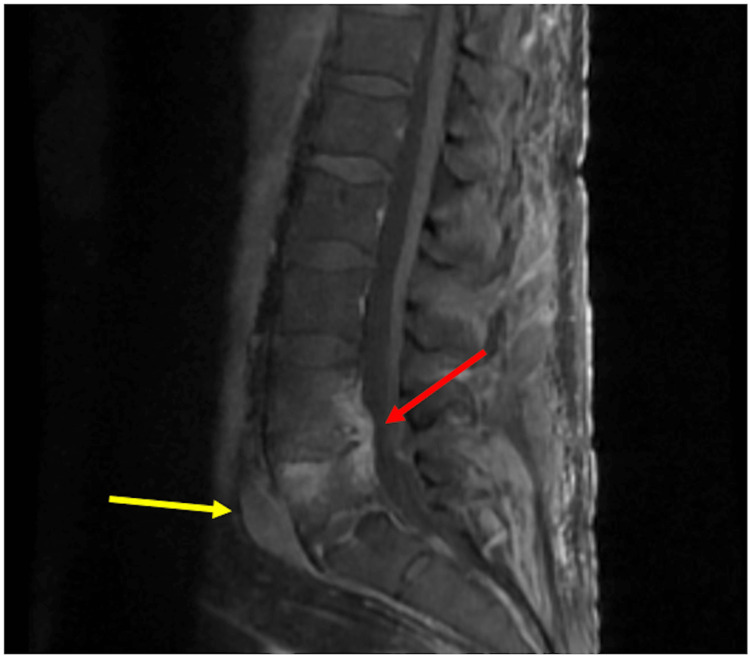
Magnetic resonance imaging of the lumbar spine showing partial destruction of the L4-L5 intervertebral disc with enhancement of the mucous pulposis (red arrow) and L5 phlegmon (yellow arrow).
